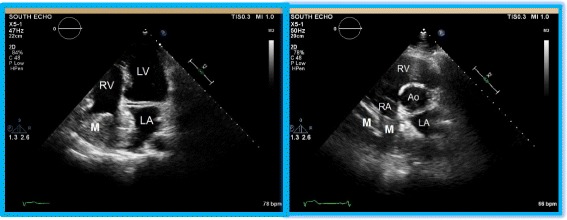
Echocardiographic imaging of the cardiac lipoma. The apical four chamber view and the parasternal short axis view clearly demonstrate the mass (M) originating from the free wall of the right atrium. The mass almost completely obliterating the right atrial cavity. Also notable is the involvement of the inter-atrial septum by this heterogenous mass. RA—right atrium; LA—left atrium; RV—right ventricle; LV—left ventricle; Ao—aorta
